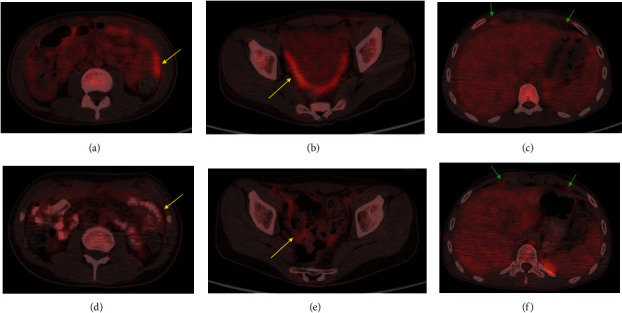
Top images (a–c): initial PET/CT demonstrating FDG avid thickening of the peritoneum and omentum (yellow arrows) and FDG avid nodules along the anterior diaphragm (green arrows). Bottom images (d–f): PET/CT 2 months later with decreased FDG avidity of the peritoneum and omentum (yellow arrows) and nodules along the anterior diaphragm (green arrows).
